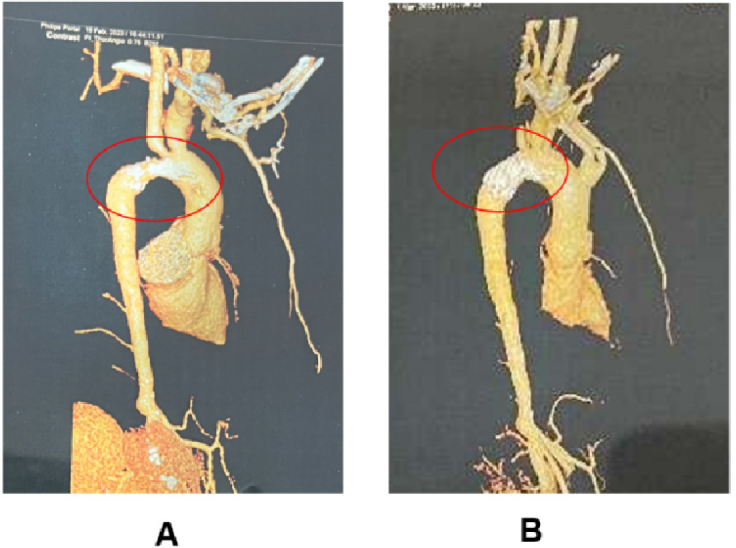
Aortic CTA of the case 1. A: Preoperative CTA of the case 1; B: Postoperative CTA of the case 1. CTA, computed tomography angiography.
